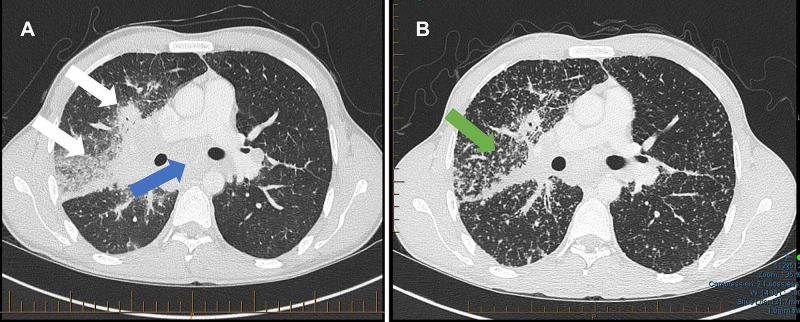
Chest CT scan A) at the diagnosis, showed right upper and middle zone parenchymal infiltrate (white arrows) and extensive mediastinal adenopathy (blue arrow); B) Worsening symptoms, showed a widespread miliary pattern (green arrow) with extensive involvement. CT - computed tomography
